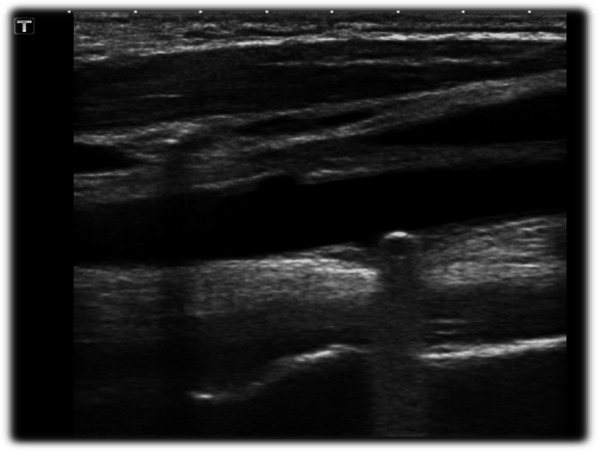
Ultrasound view of left common carotid artery demonstrating two saccular pseudoaneurysms and bullet
